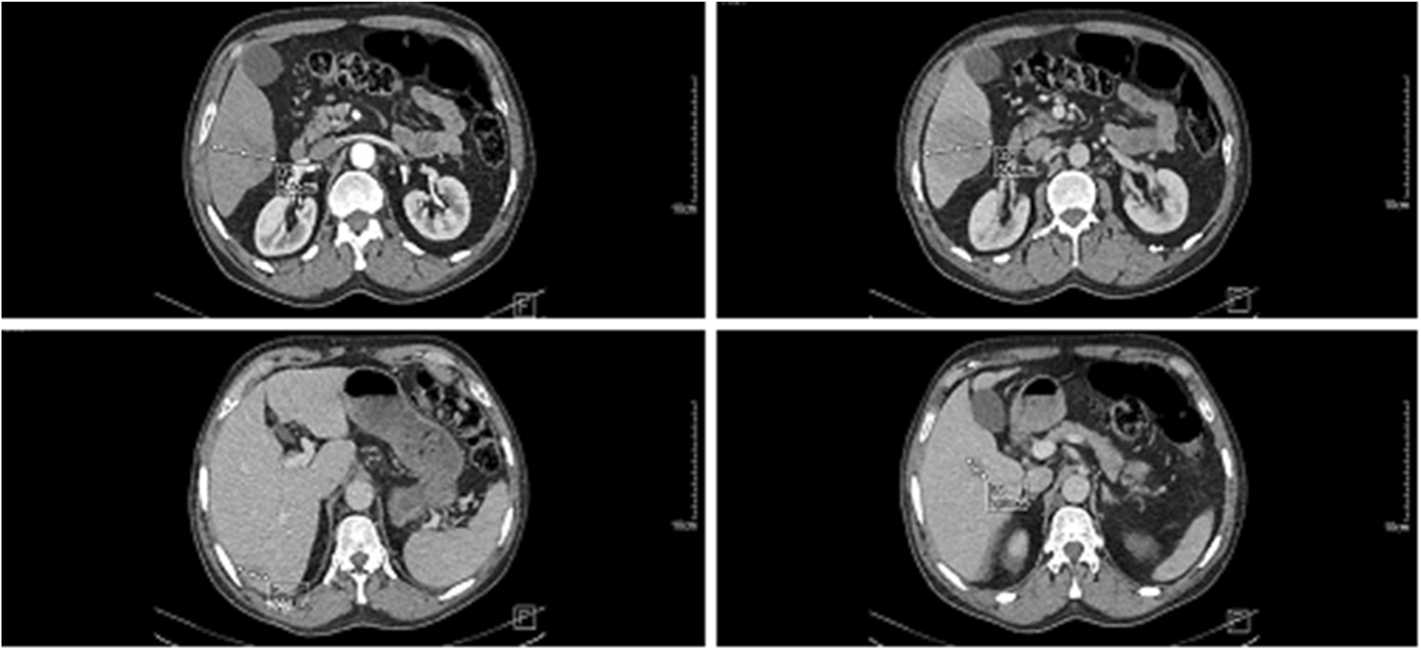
Enhanced computed tomography images showing three liver lesions of 20 mm (liver segment 7), 27 mm (liver segment 5), and 60 mm (liver segment 6)
